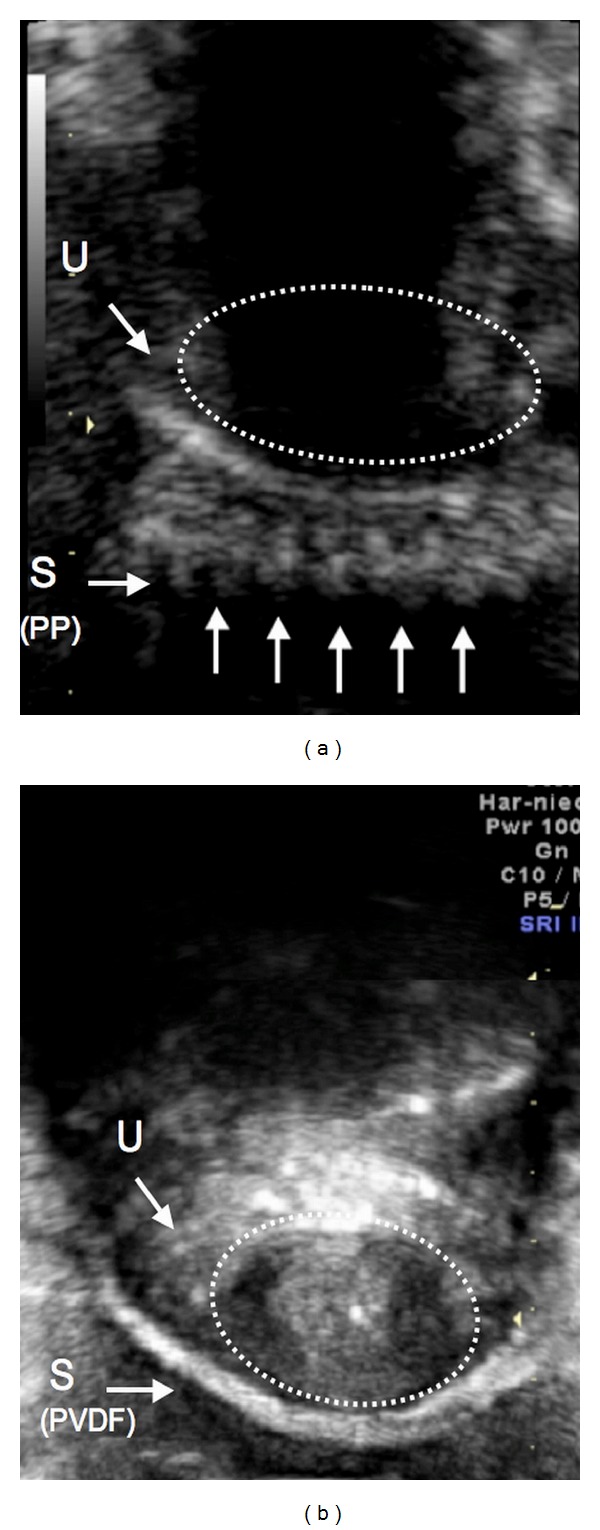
Picture from perineal ultrasound showing a PP-sling “S (PP)” with sharp pointing selvedges (arrows, (a)). A PVDF-sling “S (PVDF)” with smooth selvedges (b). Inside the dotted line: urethra (U).
